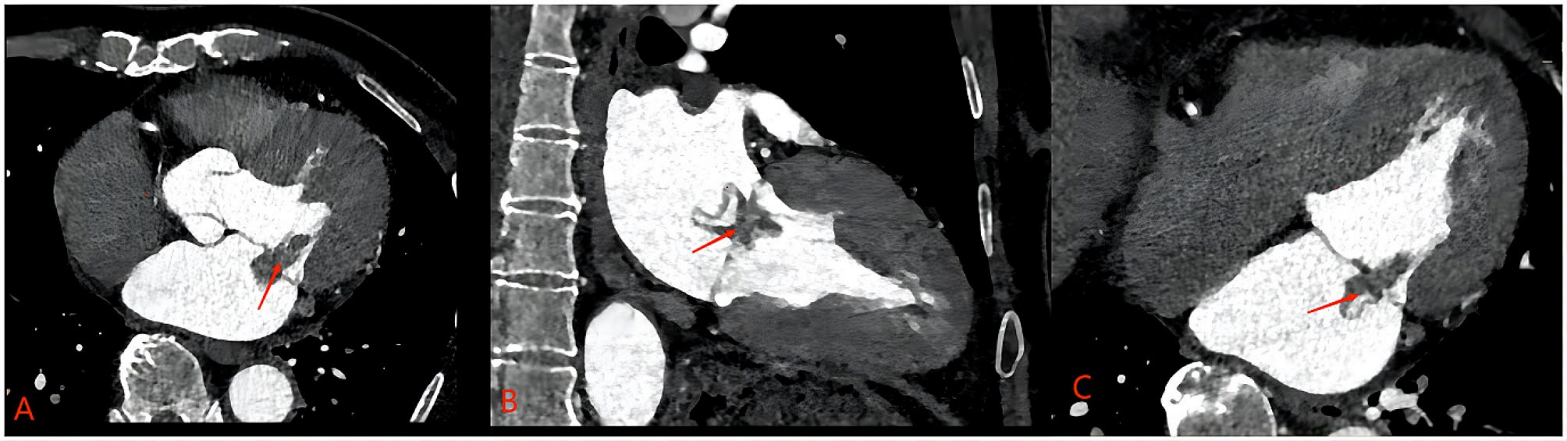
Computed tomography (CT) examination reveals an elliptical low-density filling defect in the mitral valve area with a cross-sectional size of about 2.9*1.2 cm (A,B,C) represented by red arrows
